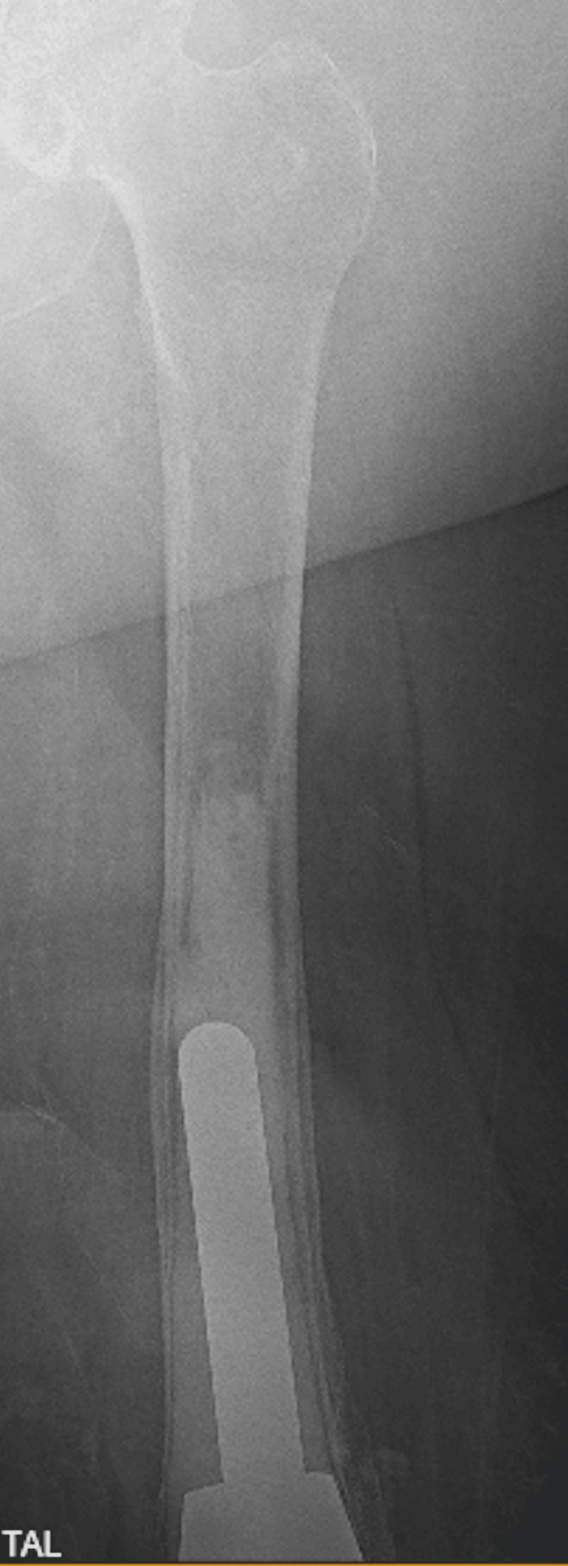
Left femur X-ray This image shows how proximal the femoral hinge joint extends into the bone.
